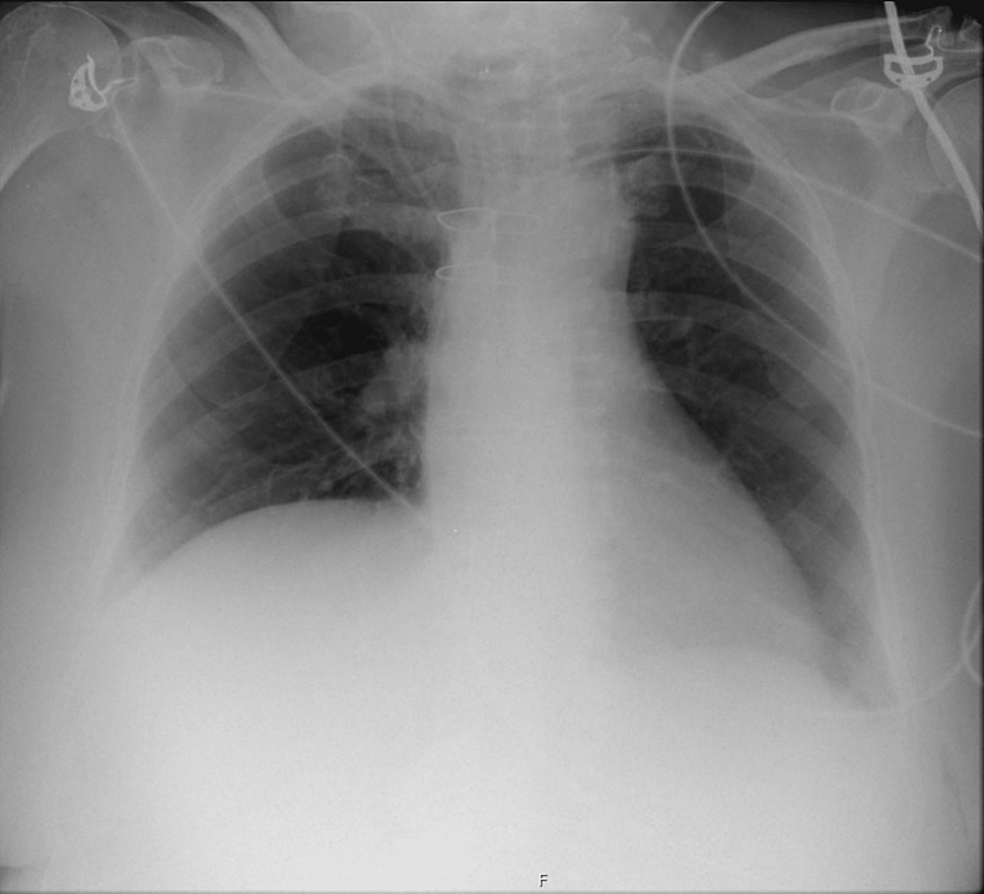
Chest x-ray at the emergency department
